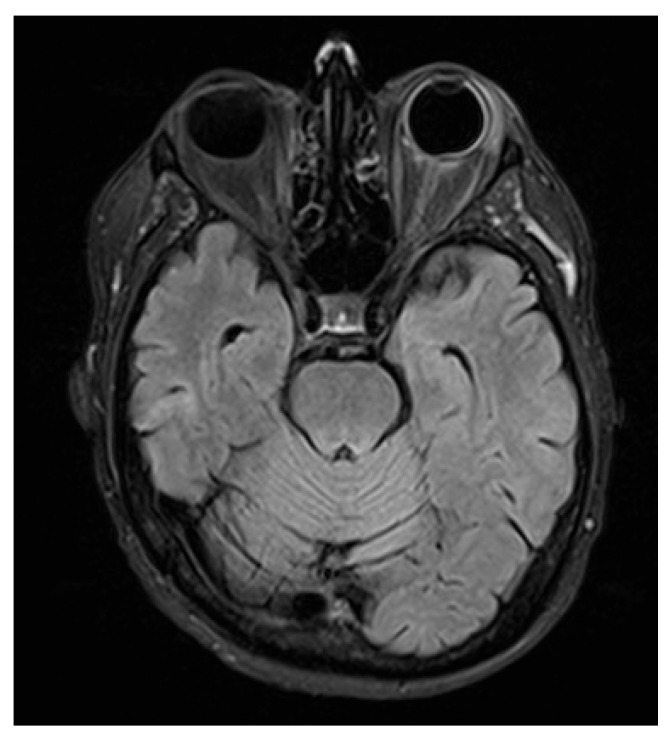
Orbital MRI (T2-FLAIR) showing hyperintensity of the retro- and periorbital fat, posterior sclera, and optic nerve sheath, consistent with leukemic infiltration extending to adnexal and retrobulbar tissues.
